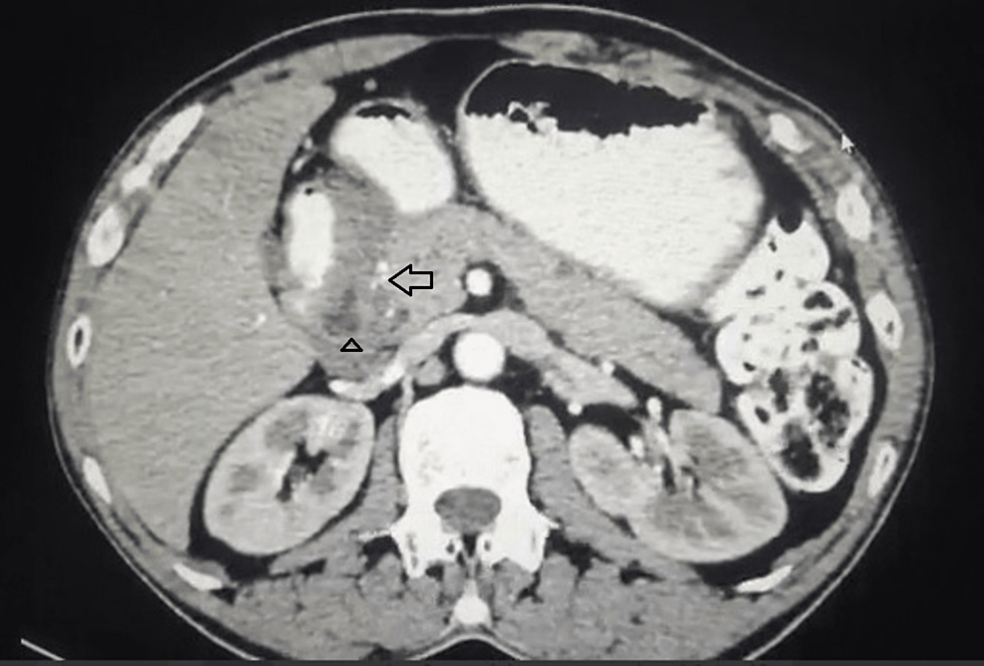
Contrast-enhanced CT scan showing a heterogeneously enhancing mass lesion involving the head of the pancreas (arrow) and the second part of the duodenum (D2) with cystic spaces (triangle)
